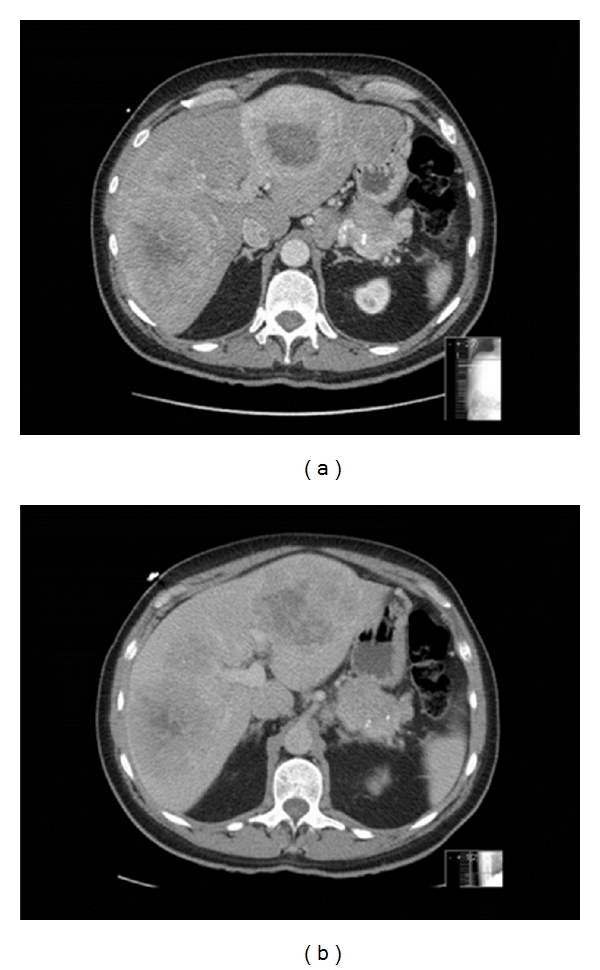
CT of bilobar hepatic metastases from a malignant NET in the (a) arterial phase and (b) venous phase. The characteristic enhancement of the tumor on arterial phase is apparent, as well as the relative darkening of the tumor on venous phase; the area of central necrosis is dark in both phases. Note the primary NET in the tail of the pancreas.
